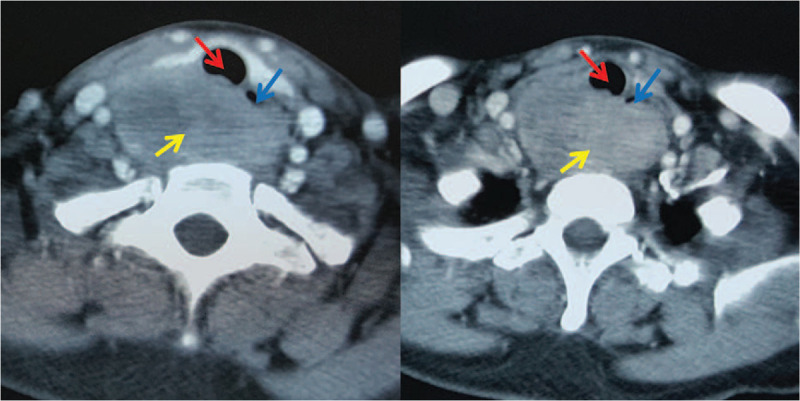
Computed tomography scan: the tumor was located between the cervical esophagus and trachea, and compressed them. Note: tumor (yellow arrow); trachea (red arrow); esophagus (blue arrow).
